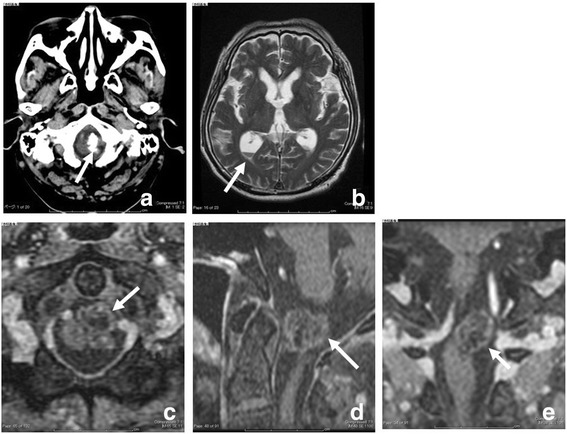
Axial computed tomography demonstrating an intradural extramedullary upper cervical calcified mass lesion (a, *white arrow*). Axial T2-weighted magnetic resonance imaging demonstrating an intraventricular hemorrhaging (b, *white arrow*). Axial (c), sagittal (d) and coronal (e) T1-weighted contrast-enhanced magnetic resonance images demonstrating an intradural extramedullary mass lesion near the left C1 root which compressed the spinal cord (*white arrows*)
